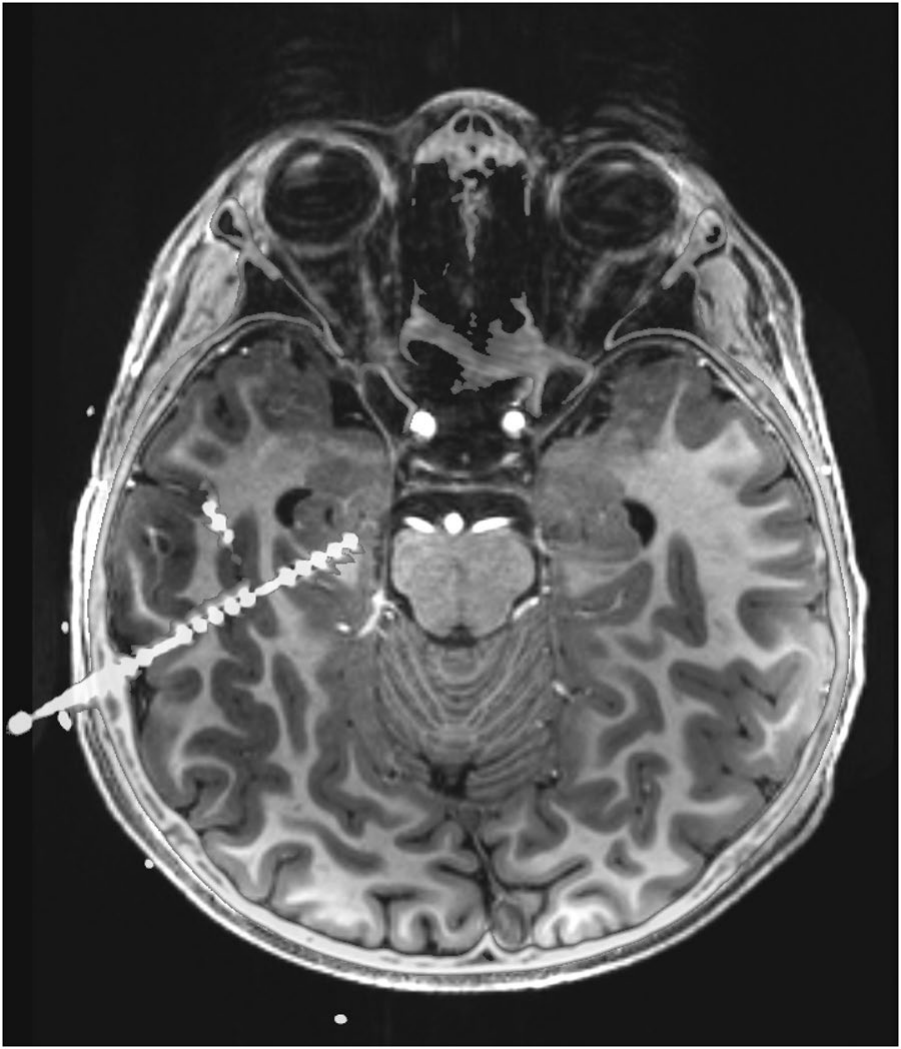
A patient undergoing invasive EEG monitoring with stereotactic depth electrodes had a high-resolution spiral CT image acquired following electrode placement for verification and to rule out hemorrhage. The CT images were co-registered to the patient’s pre-operative 7-Tesla T1 weighted MRI using MIM version 6.8.3 (Mim Software Inc.), with pixel intensities averaged to optimize concurrent visibility of CT electrodes and MRI tissue contrast.
